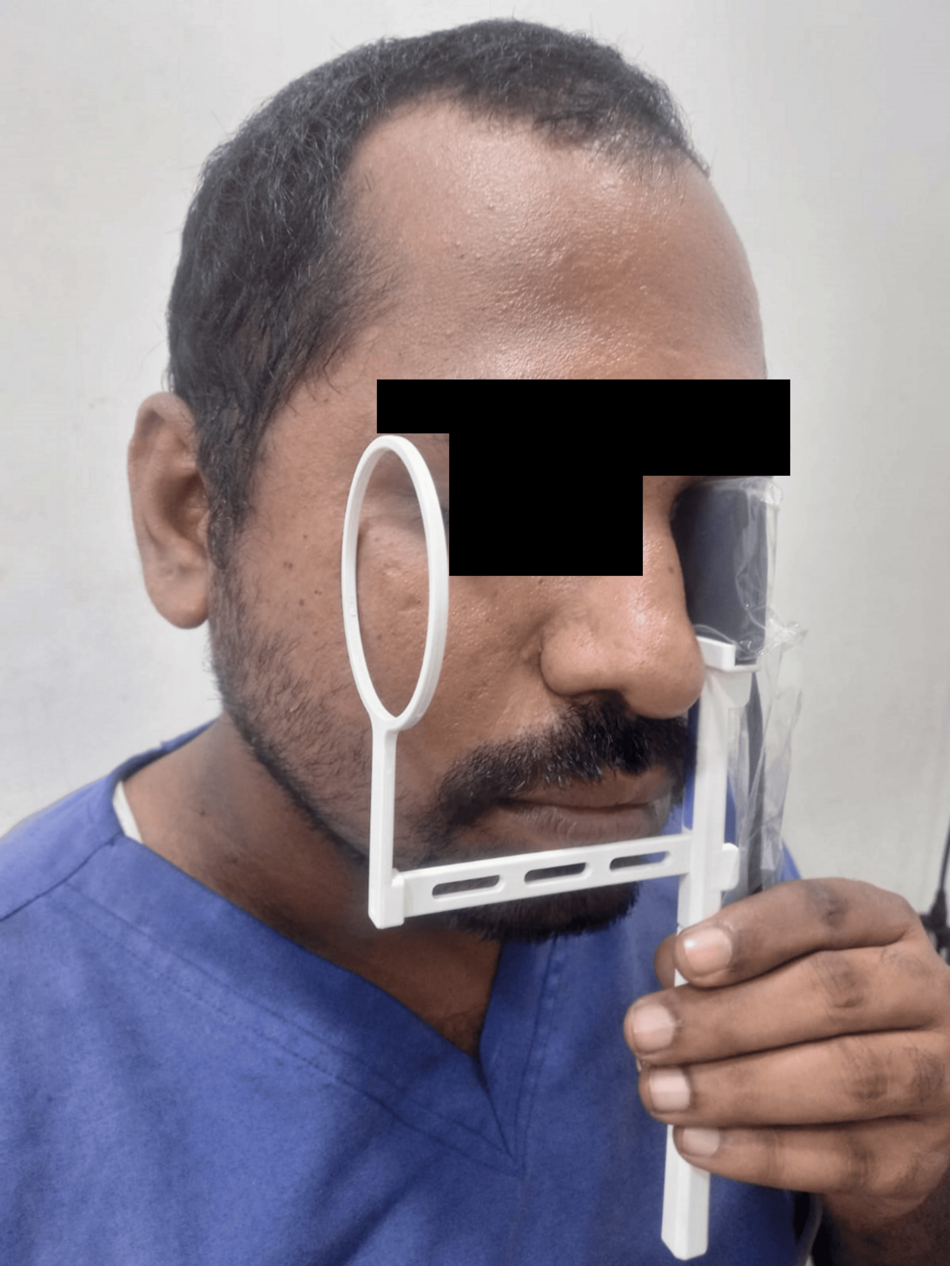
RVG sensor with the holder in the position for nasal bone radiography The figure shows the RVG sensor mounted on a custom-designed external holder and positioned over the nasal bridge. This standardized setup ensures optimal and reproducible alignment of the sensor for capturing clear radiographic images of the nasal bone anatomy during intraoperative or clinical assessment. RVG: radiovisiography
